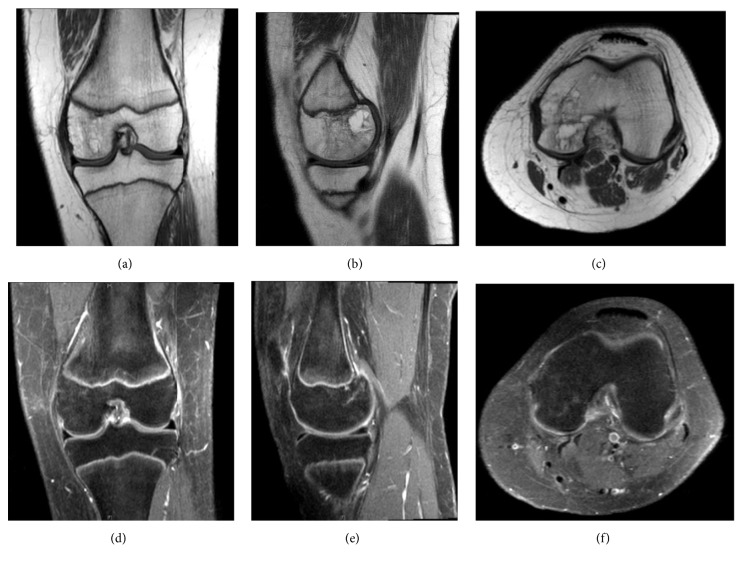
Magnetic resonance images (MRI): T1 coronal, sagittal, axial views (a, b, c) and T1 fat saturated w/contrast coronal, sagittal, axial views (d, e, f) of the left knee after completion of chemotherapy, October 2010. Near complete resolution of lesion.
